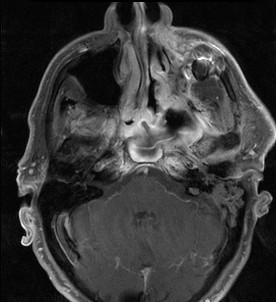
1st folow-up 6 weeks post completion of RT: complete remission with only posttherapeutic changes: axial, contrast enhanced T1 weighted MRI.
